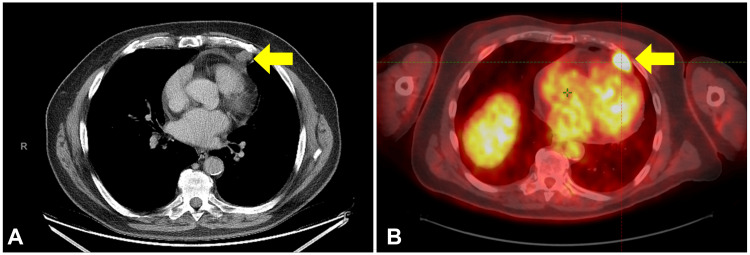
Diagnostic imaging. (A) Chest CT scan and (B) PET CT showing the asymptomatic pericardial nodule (yellow arrows). CT = computed tomography, PET = positron emission tomography
